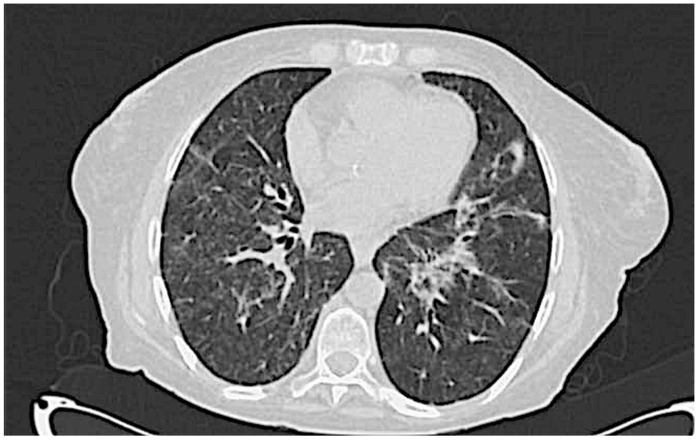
First HRCT scan showing interstitial involvement characterized by widespread thickening of inter- and intra-lobular septa centrally and peripherally in both lungs, along the pleural surfaces and fissural planes. This is accompanied by multiple areas of increased density with a ground-glass appearance.
